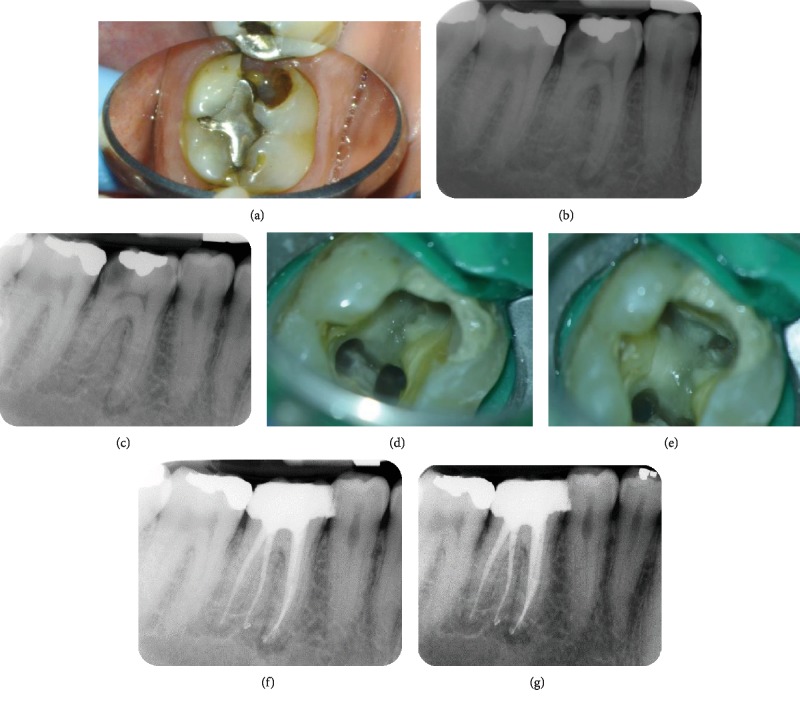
(a) Intraoral photo of tooth #46 showing a large carious lesion and defective amalgam restoration. (b, c) Periapical radiographs of different angles showing the additional root. (d, e) Access cavity preparation with four root canals located. (f, g) Final periapical radiographs from different angles.
